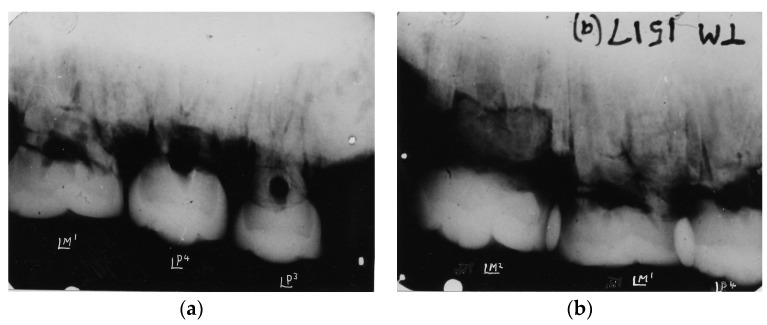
(a) Kromdraai Paranthropus TM 1517(a). Radiograph of left premolar and molar maxillary teeth reveal the graded wear of the premolars and M1; (b) Kromdraai Paranthropus TM 1517(a). Radiograph of left maxillary second premolar and maxillary M1 and M2 revealing heavy wear distally on the premolar and occlusal surface of M1 and slight wear mesially on M2.
